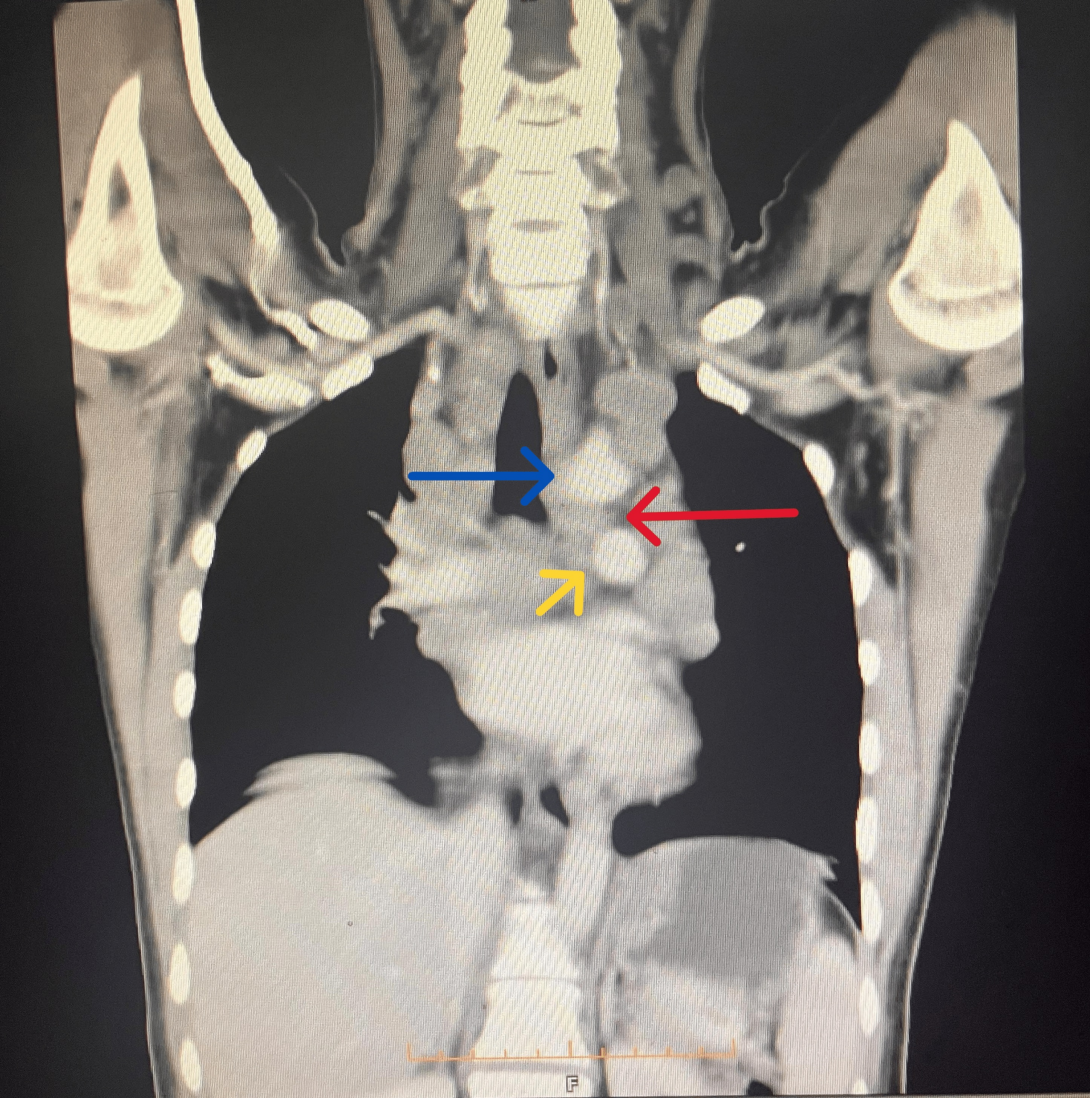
CT chest with contrast showing mediastinal lymphadenopathy. The blue arrow points to the aortic arch and the origin of the left subclavian artery, the yellow arrow points to the left pulmonary artery and the red arrow shows the lymph node present at the aortopulmonary window, likely compressing the left recurrent laryngeal nerve as it swings around the aortic arch. CT: Computerized tomographic scan.
